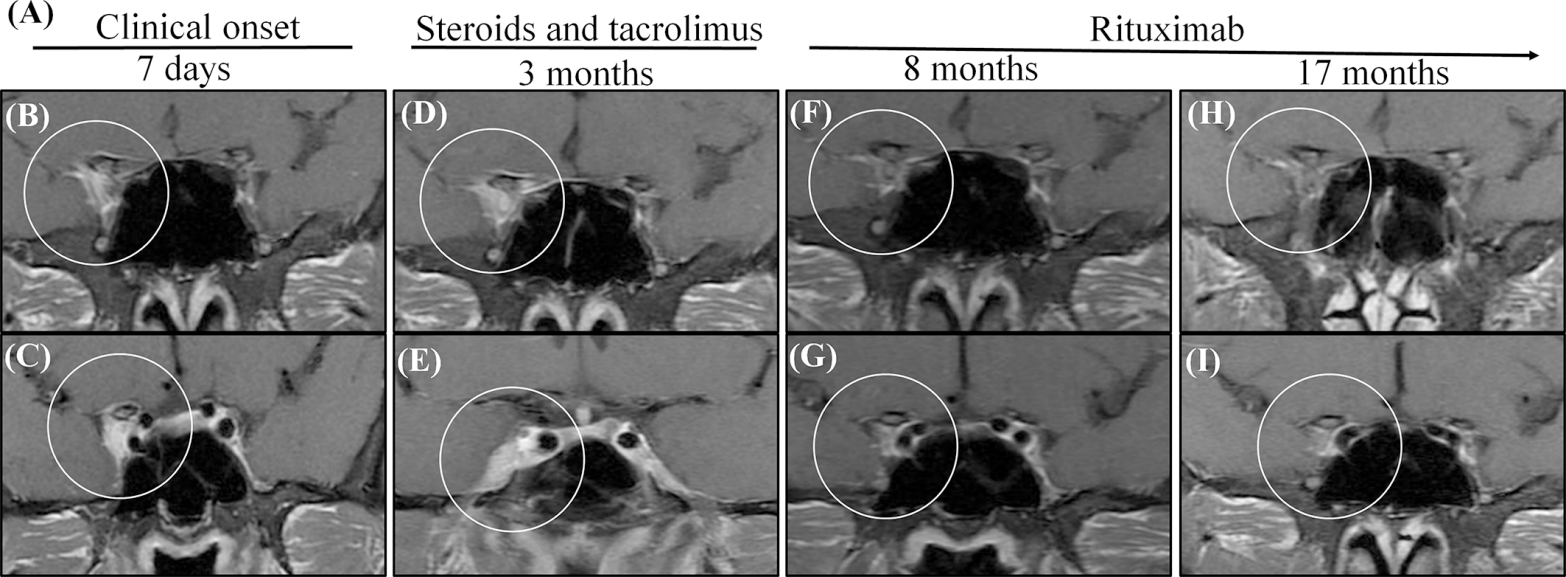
MRI shows that administration with steroids and tacrolimus was not successful for patient 1, while administration with RTX. (A) The summarized clinical course and therapy of the patient. (B, C) Initial MRIs showed a heterogeneous contrast-enhanced mass in the right cavernous sinus (circle). (D, E) Two times consecutively, the symptoms relapsed when prednisone was reduced to 40 mg per day although he oral tacrolimus at the same time. The MRIs obtained after 3 months reveal an enlarged size and enhancement of the cavernous sinus; the dura was involved [circle in (E)]. (F, G) After changing tacrolimus to RTX 5 months, in the MRIs obtained 8 months after the onset, the cavernous lesion disappeared (circle). (H, I) After stopping prednisone for 1 year and RTX for half a year, there was still no recurrence 17 months after the onset, and there were still no lesions in the cavernous lesion.
